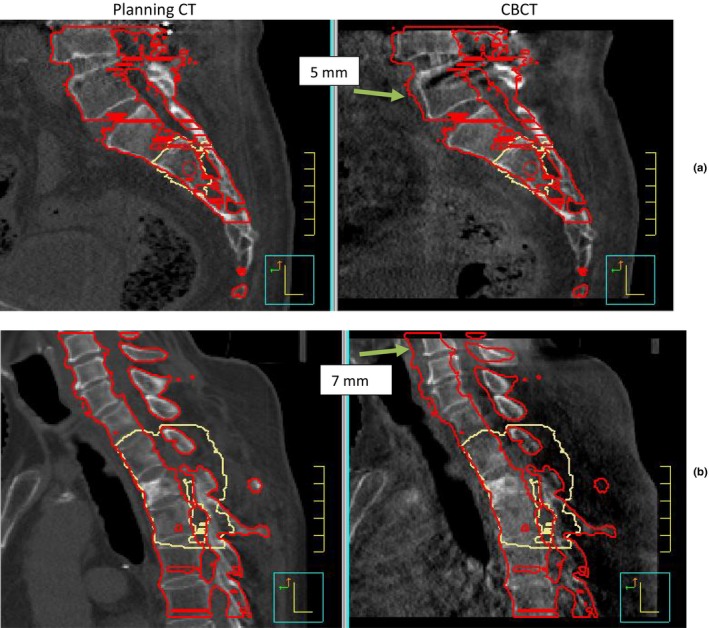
Sagittal view of case # 45 (a) and # 10 (b). Red and yellow are contours of vertebrae and targets in planning CT. Large residual errors due to curvature change at L to S transition (a) and C to T transition (b) are marked with arrows.
